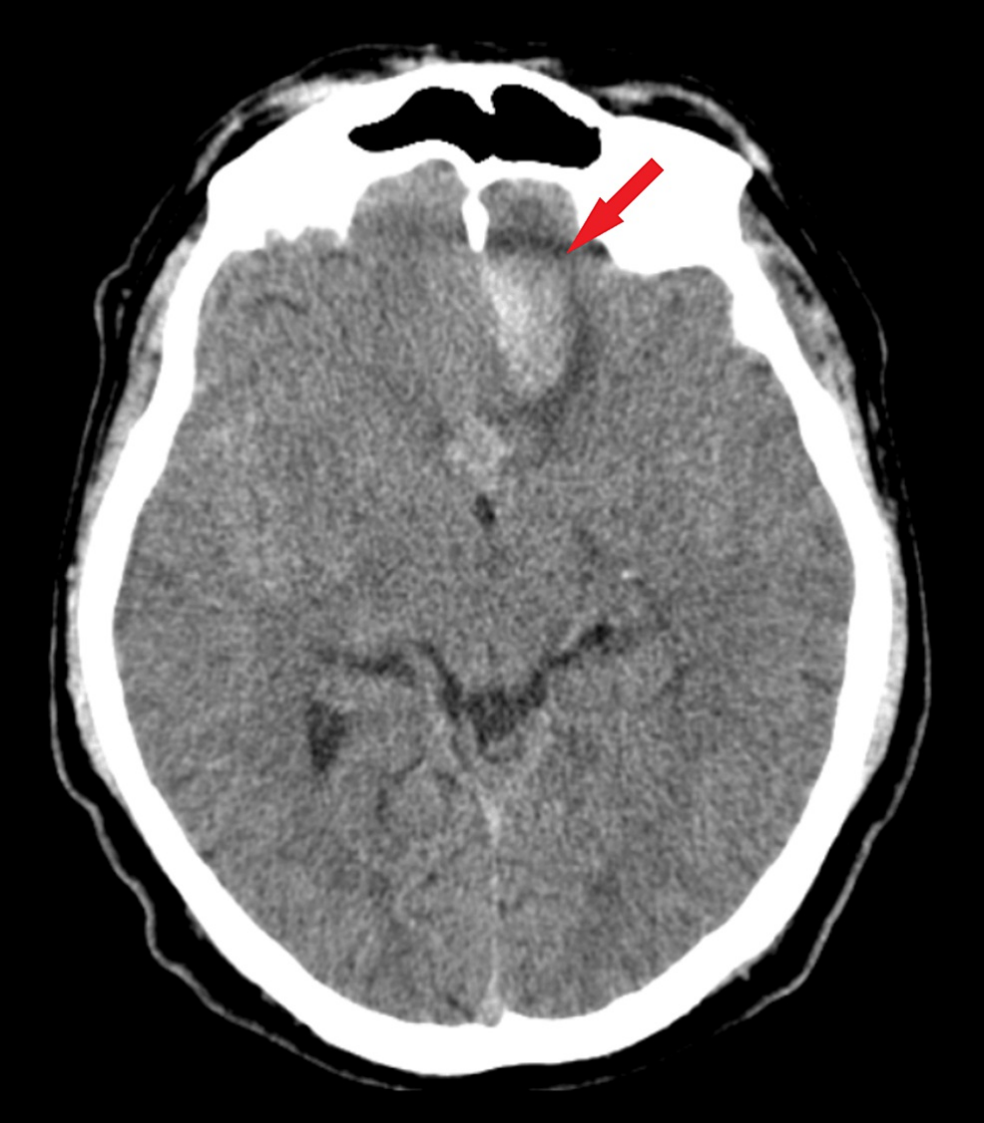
Preoperative CT scan. The axial section of the CT scan highlights a frontobasal hematoma with perilesional edema (red arrow).
